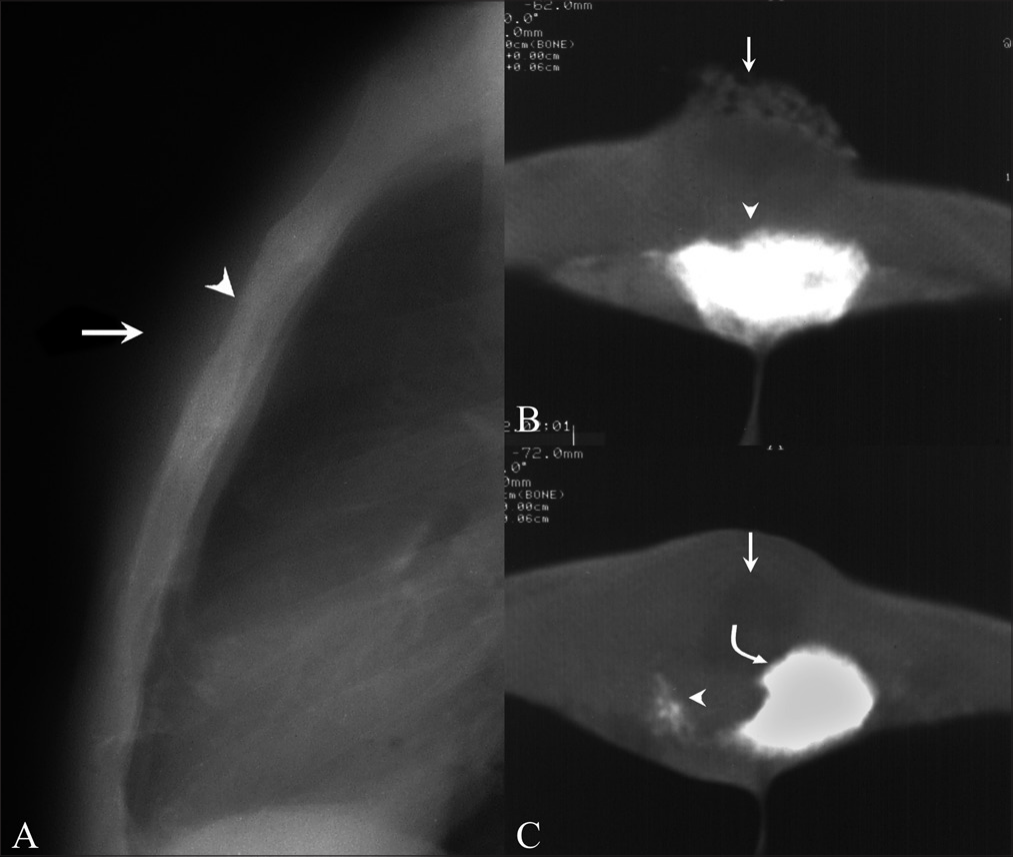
Sternal tuberculosis – patient 2. Lateral radiograph (A) of the sternum shows an osteolytic lesion (arrowhead) with overlying soft tissue swelling (arrow). CT scans (B, C) show dense sclerosis of the sternal body (arrowhead in B) associated with a large skin ulcer (arrow in B) with osteolysis (arrowhead in C) more caudally, with sclerosis of the remaining fragment (curved arrow in C) and an adjoining soft tissue abscess (arrow in C)
